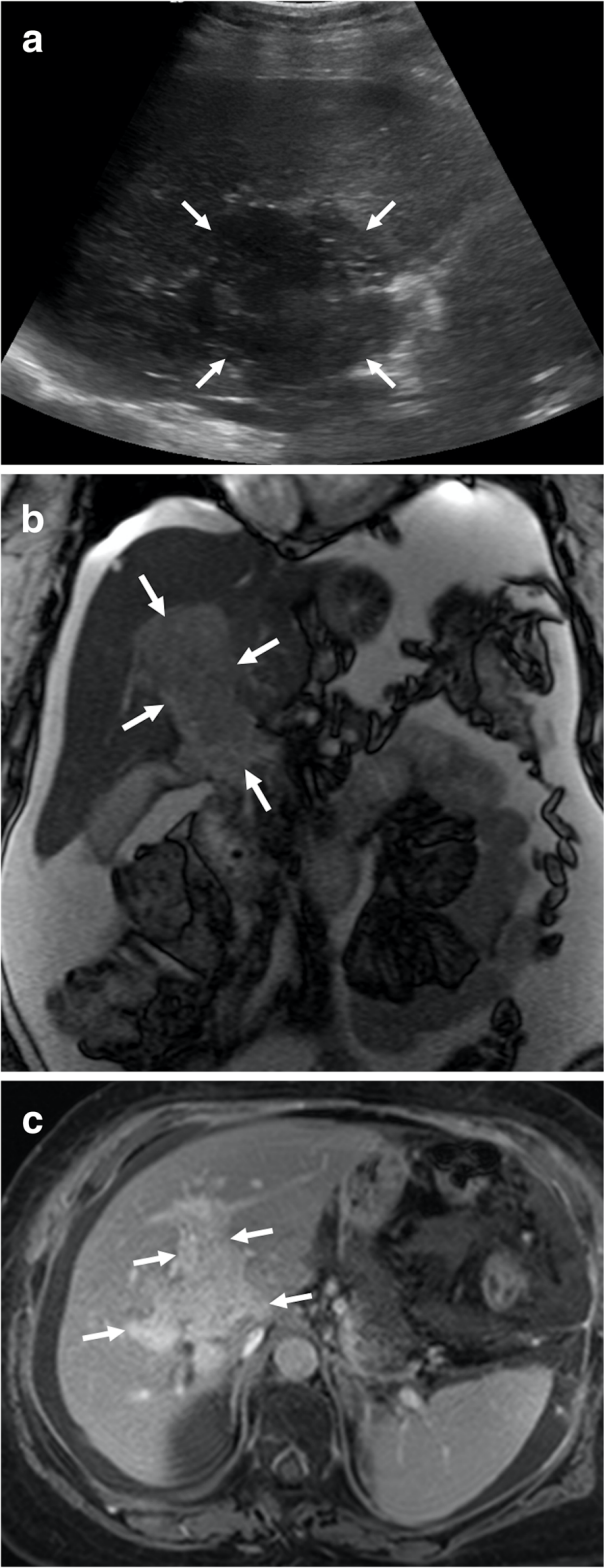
A 62-year-old female with unremarkable medical history now presents with right upper quadrant pain and weight loss. a Abdominal US study shows a large, hypoechoic solid mass with ill-defined borders (arrows) at the liver hilum. b Coronal T2W MR image demonstrates an infiltrative, expansile mass within the main portal vein and its distal branches (arrows). c Axial plane postcontrast equilibrium phase T1W MR image demonstrates intense contrast enhancement of the mass (arrows) suggestive for a tumor thrombus over a bland thrombus. There were no associating imaging findings suggestive for parenchymal chronic liver disease or parenchymal HCC focus. Percutaneous image guided biopsy revealed portal vein leiomyosarcoma
